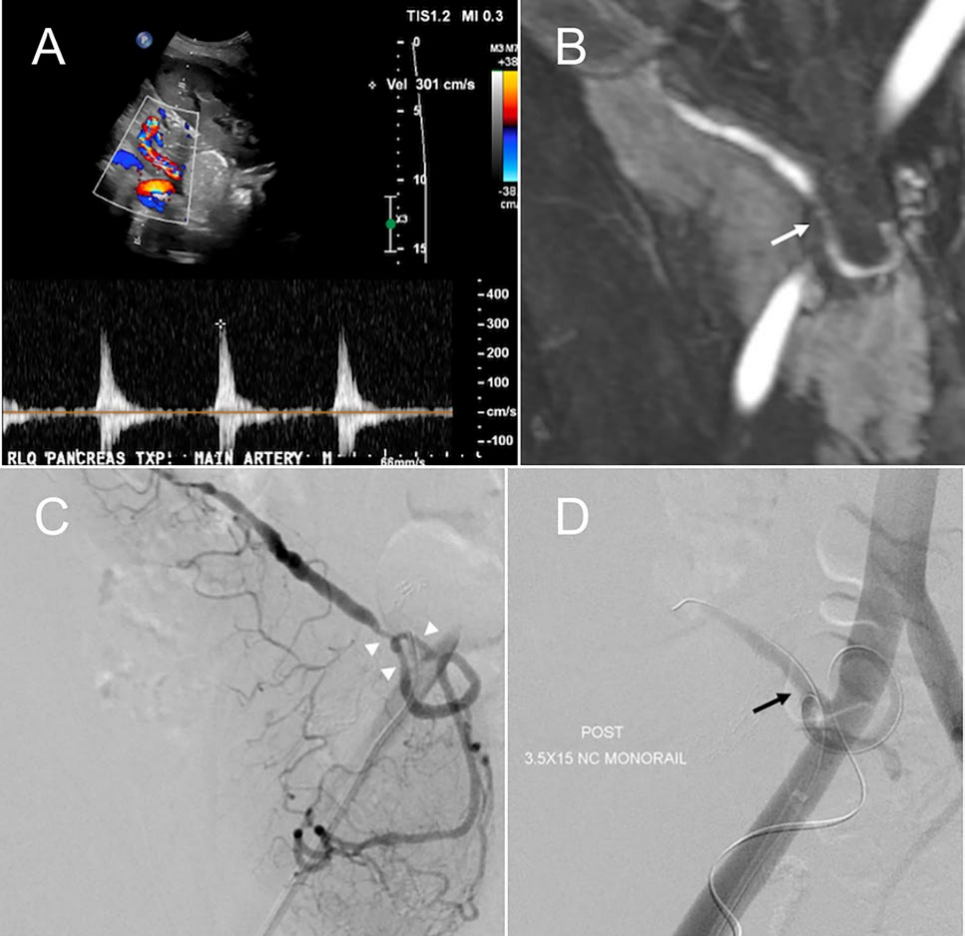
Arterial stenosis. A 51-year-old male status post simultaneous pancreas and kidney transplant for type 2 diabetes mellitus and diabetic nephropathy. Spectral Doppler ultrasound (A) performed at 3 months shows persistently elevated peak systolic velocity of 301 cm/s with turbulent flow at the anastomosis. Magnetic resonance angiography confirms the finding of stenosis at the anastomotic site (white arrow, B). Conventional angiography (C) also confirms the stenosis at the anastomosis (arrowheads). Post-balloon angioplasty angiography (D) shows improvement of the stenosis (black arrow)
